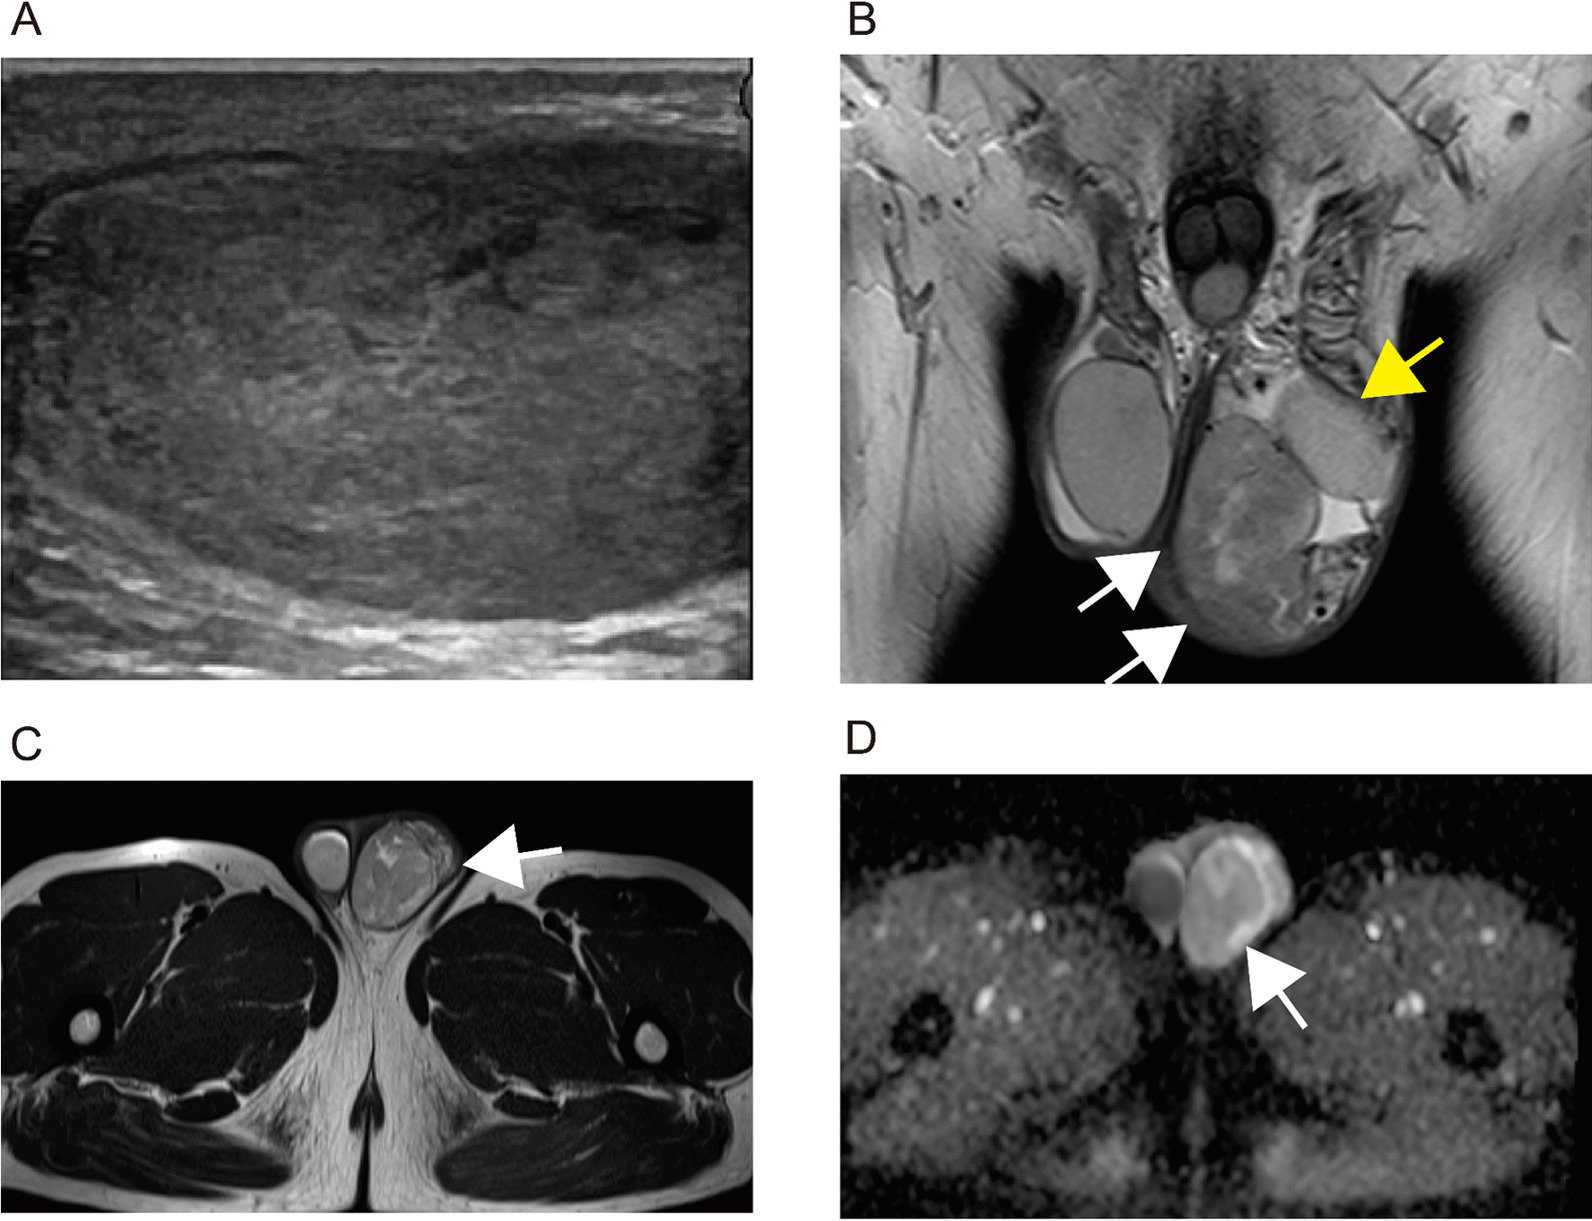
Ultrasound and magnetic resonance imaging. A Ultrasonography showed internally hypoechoic heterogeneous mass, 5.5-cm-long diameter in size. The tumor showed a clearly demarcated solid mass. B, C T2 weighted images by magnetic resonance imaging examination revealed heterogeneous low intensity mass (white arrows) including focal high intensity areas (B coronal imaging, C axial imaging). Left testis was confirmed (yellow arrow). D Diffusion-weighted imaging shows no apparent decreasing ADC value in the internal area of the mass; however, this is focally observed in peripheral area (white arrow)
